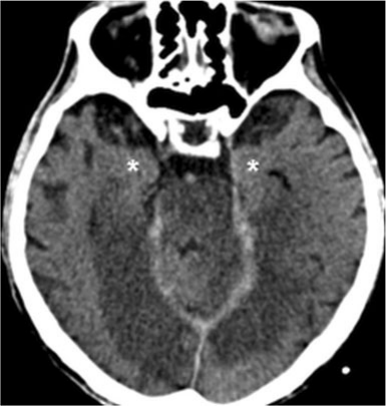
An 83-year-old male who presented with acute coma. Noncontrast CT obtained 2 days after admission shows hypodensity in the medial aspects of both temporal lobes, compatible with posterior circulation ischemia. Occipital lobes, brainstem and cerebellum are also involved, and there is relative hyperdensity of the basilar artery . Note preservation of the entorhinal cortex (*asterisks*), supplied by MCA and anterior choroidal artery branches
